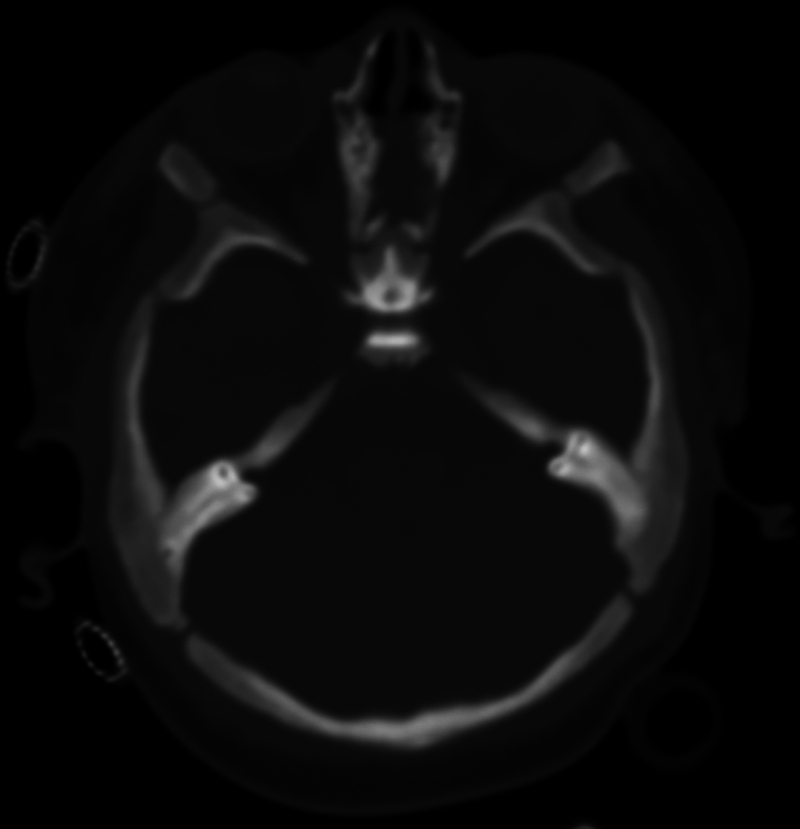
Computed tomography brain showing decompressed optic canal on the right on postoperative day 9.
